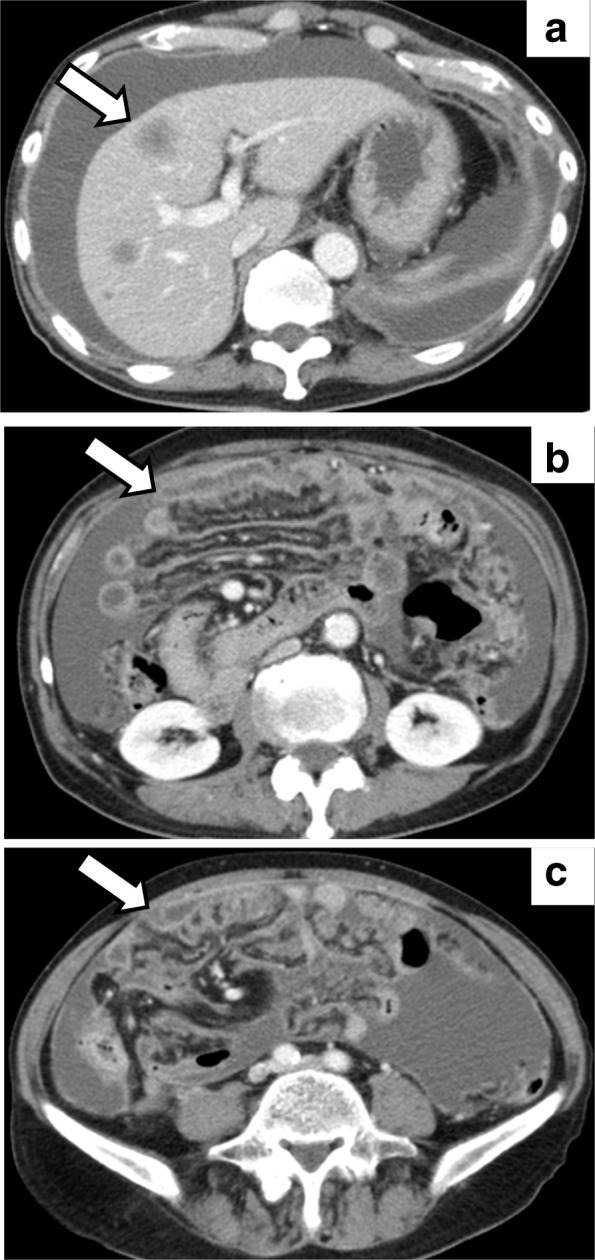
Enhanced computed tomography (CT) imaging. a After a single administration of pembrolizumab, the size of the liver metastasis increased from 1.3 to 1.6 cm (arrow). b and c Widespread peritoneal dissemination (arrows) and a large amount of ascites were visible after pembrolizumab treatment
